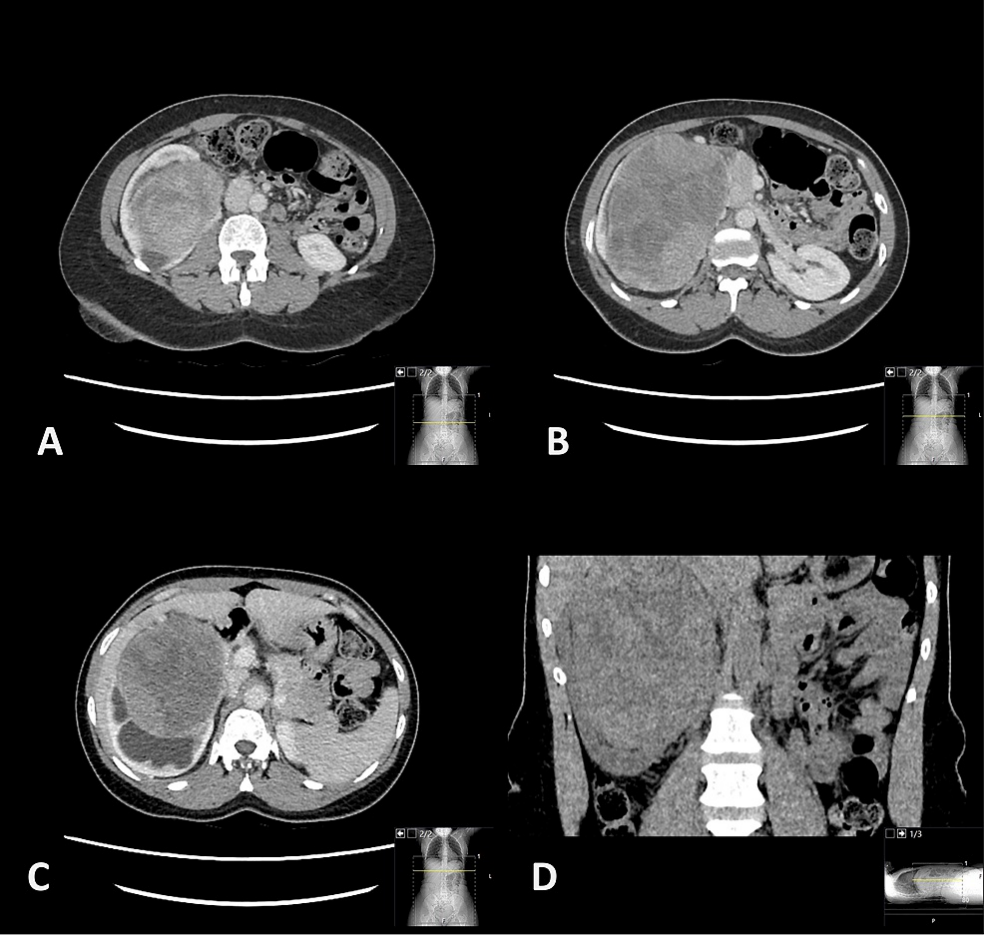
Pre-operative CT images demonstrating the large 14 cm right renal mass. (A-C) Axial and (D) coronal slices are shown. The liver was reported as clear of metastasis on CT. CT: computed tomography
